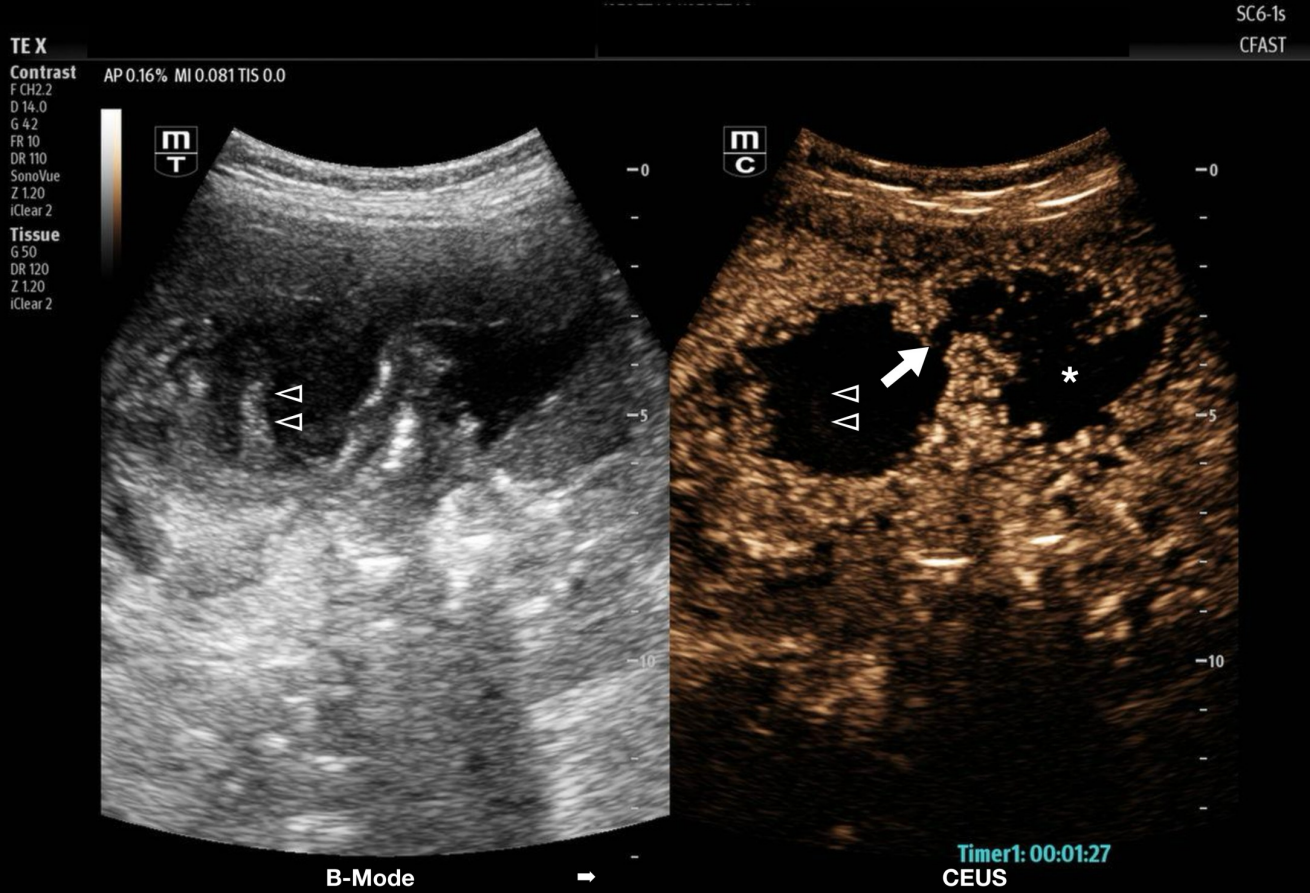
Contrast-enhanced ultrasound B-mode reference image is shown on the left (B-mode), and contrast-enhanced ultrasound (CEUS) image on the right. The CEUS image demonstrates perforation of the gallbladder wall (white arrow) with an adjacent non-enhancing fluid collection. The internal luminal echoes seen on B-mode ultrasound (US) (triangles) are unenhanced consistent with debris in the lumen.
